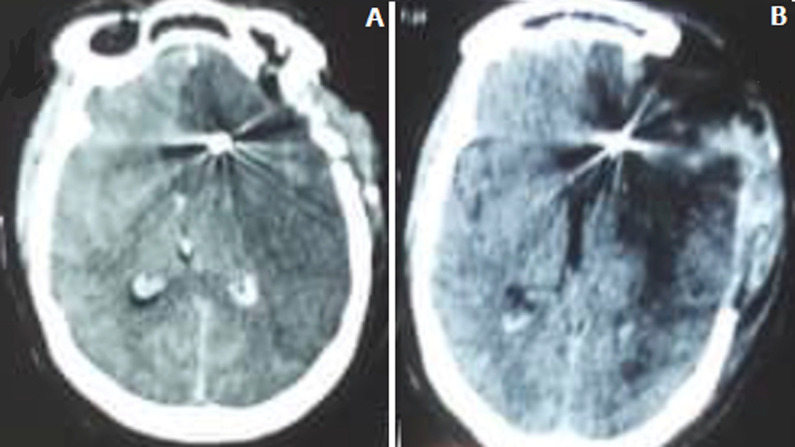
A) cerebral CT scan in preoperative axial section of a massive left sylvian infarction after clipping a carotid aneurysm; B) decompressive post-craniectomy brain CT scan of left massive sylvian infarction after aneurysm clipping in the same patient
